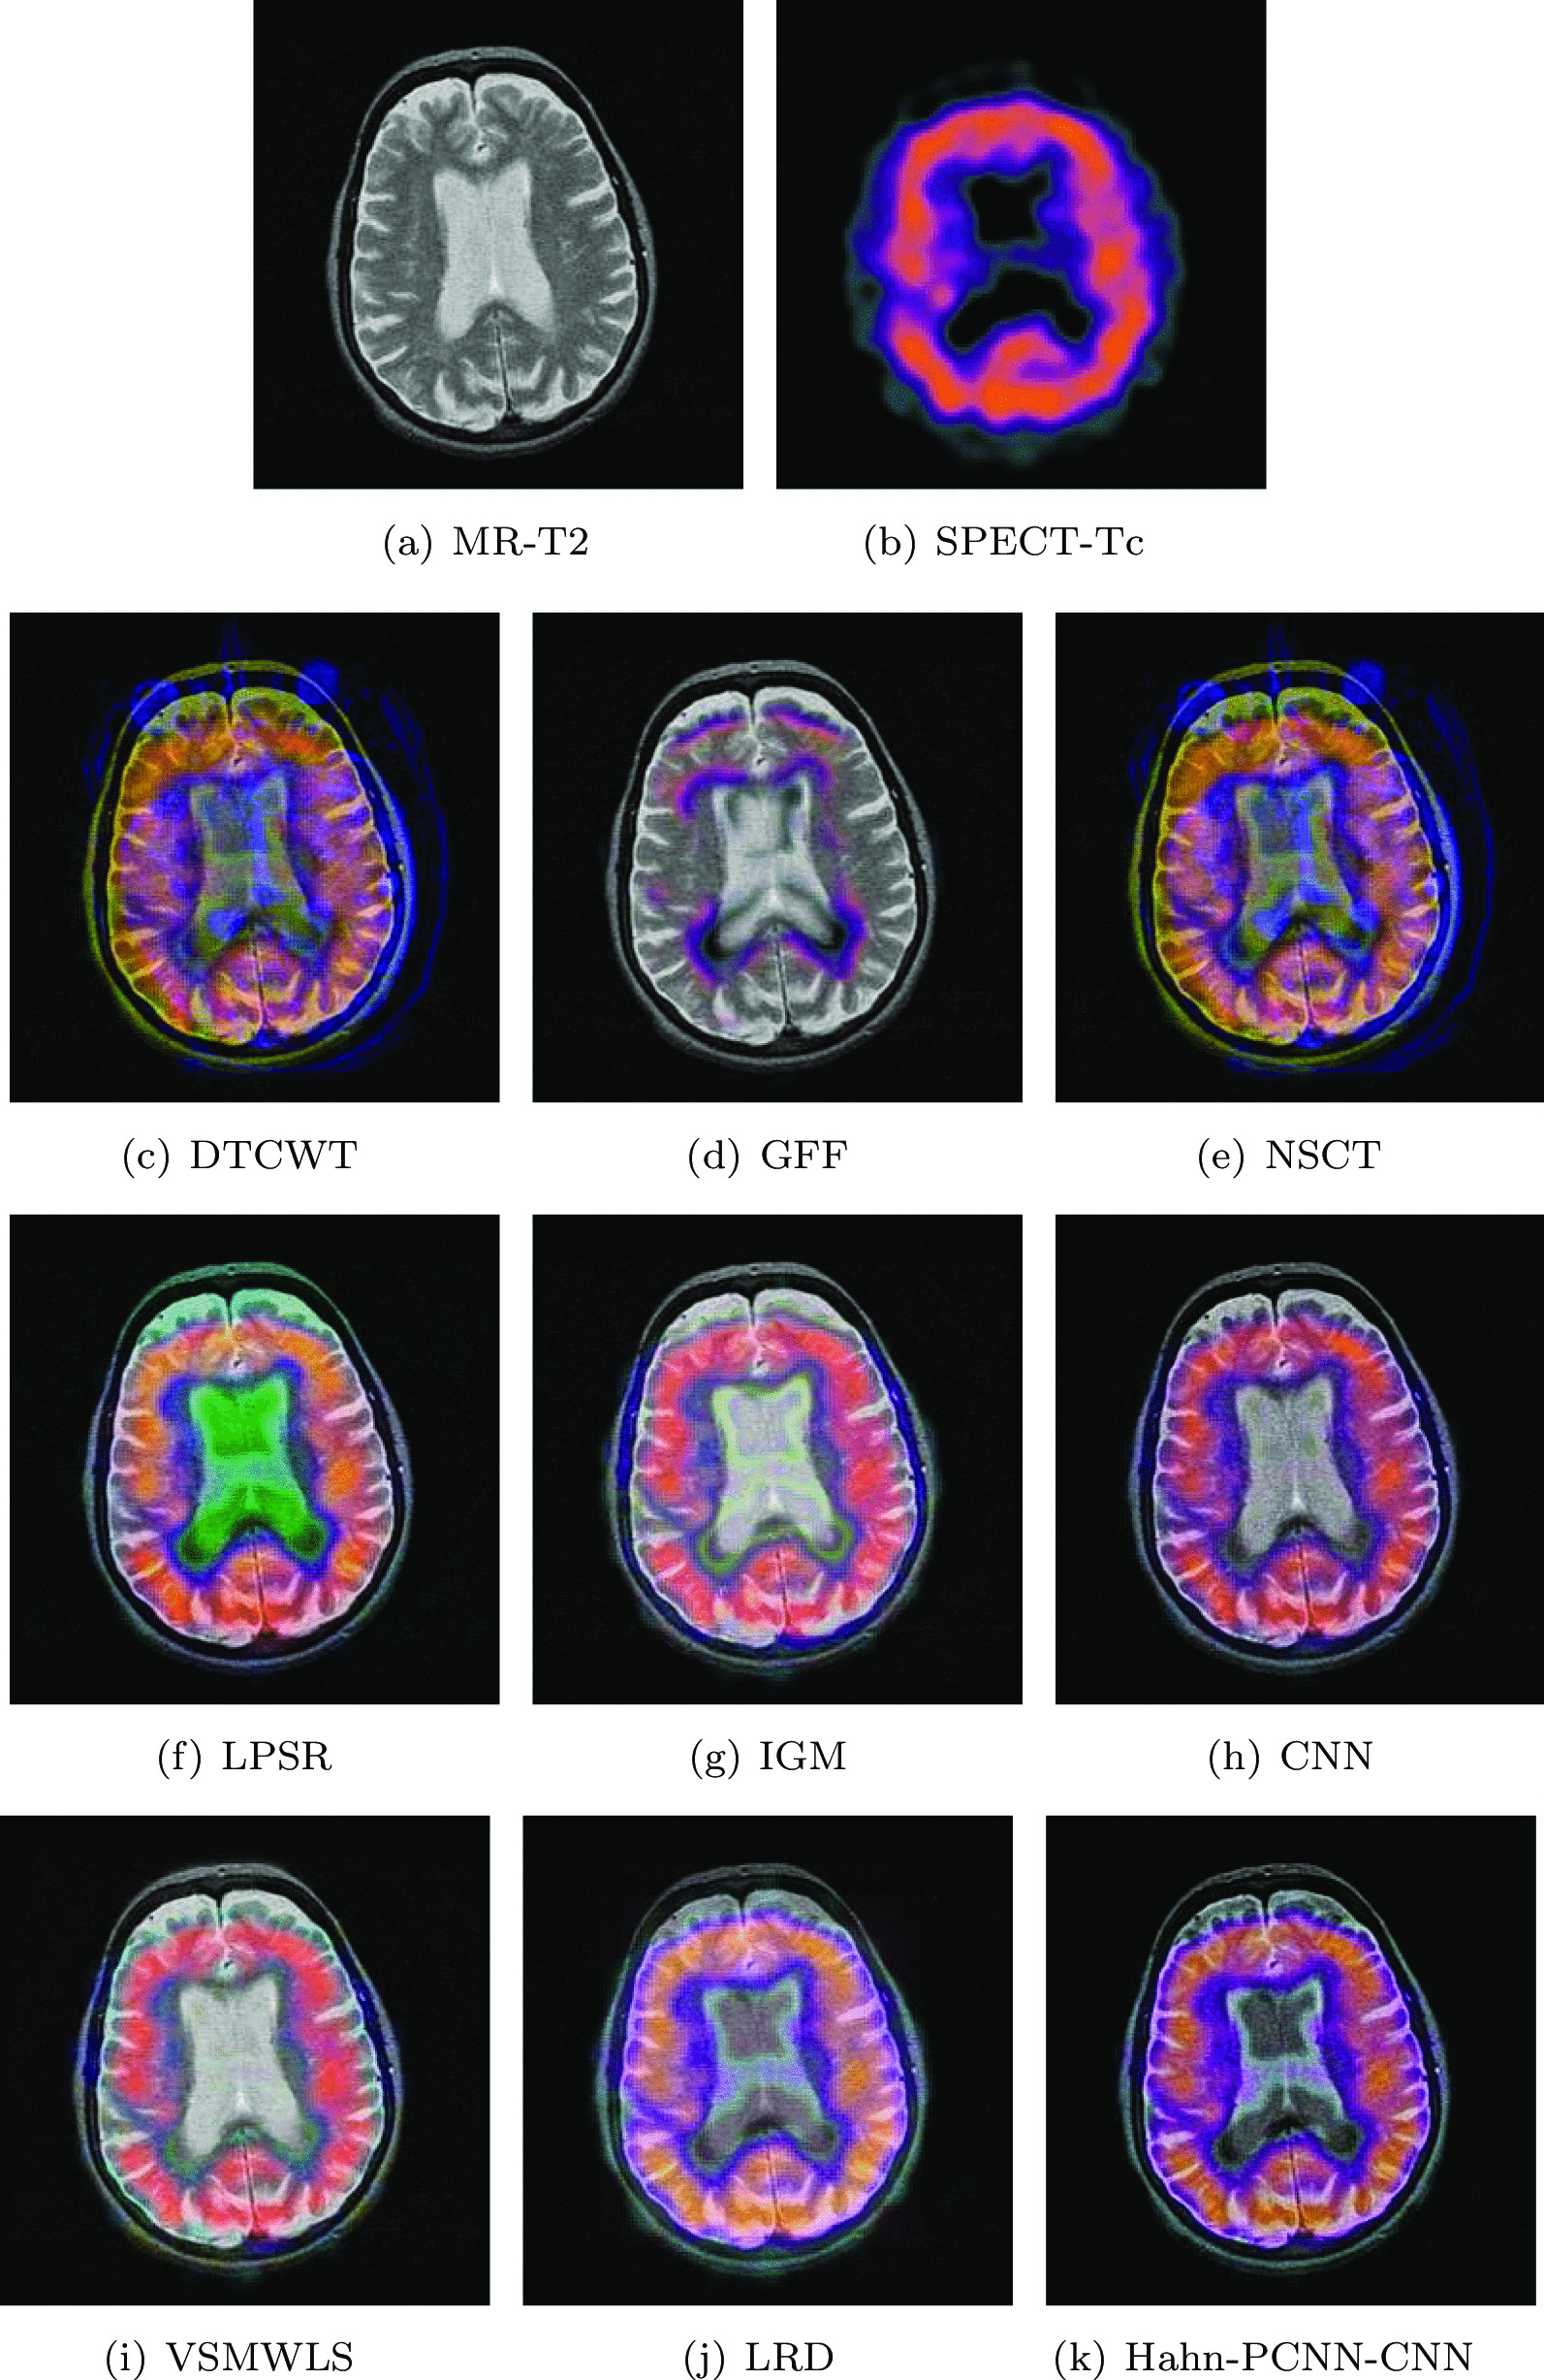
The first set of fused MRI-SPECT images from 9 methods on huntington’s disease
